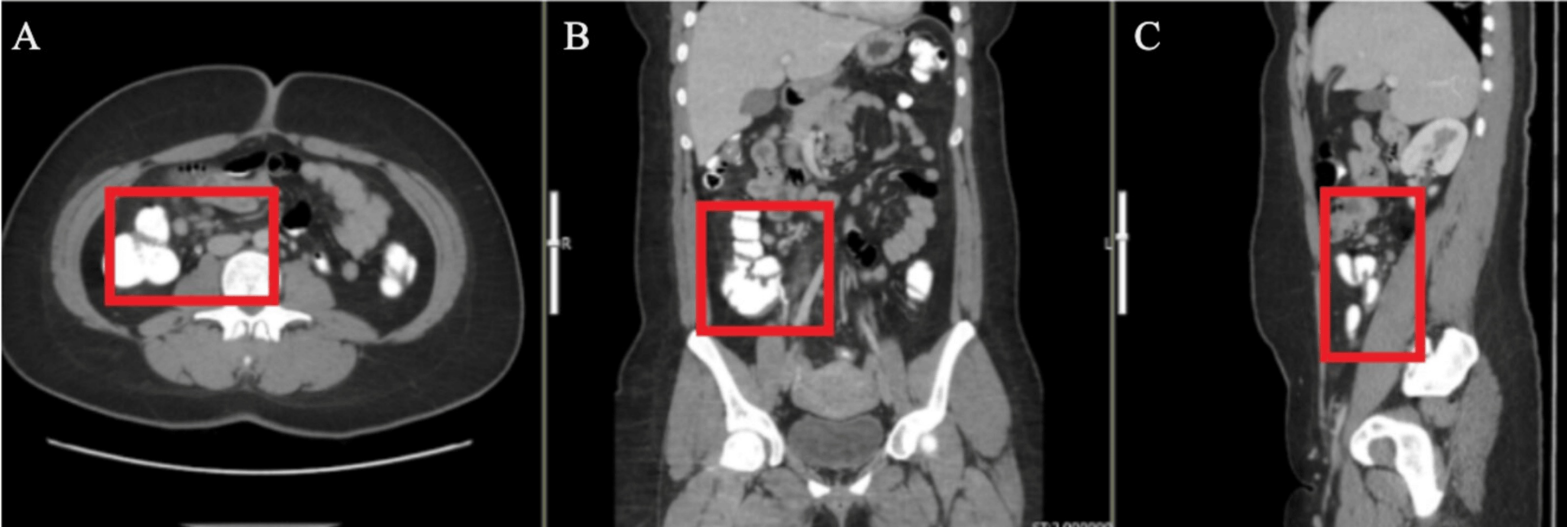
A CT scan showing the axial (A), coronal (B), and sagittal (C) views Status post appendectomy with surgical staple line, mild thickening of the cecal wall, multiple prominent lymph nodes, largest 2.1 cm x 1.5 cm with shotty subcentimeter retroperitoneal lymph nodes.
